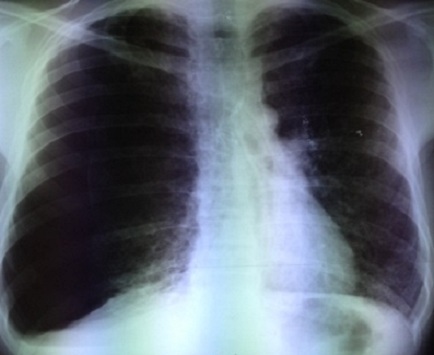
Radiographie thoracique montrant un pneumothorax total droit sur poumon kystique
